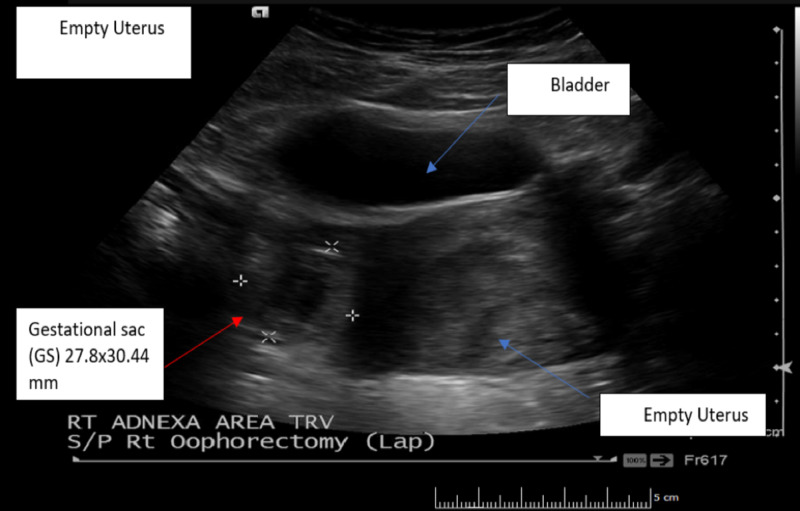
Pelvic ultrasound (transverse view): showing full bladder, empty uterus with endometrial lining thickness of 17 mm, and a gestational sac (GS) in the right adnexa measuring 27.8x30.44 mm
